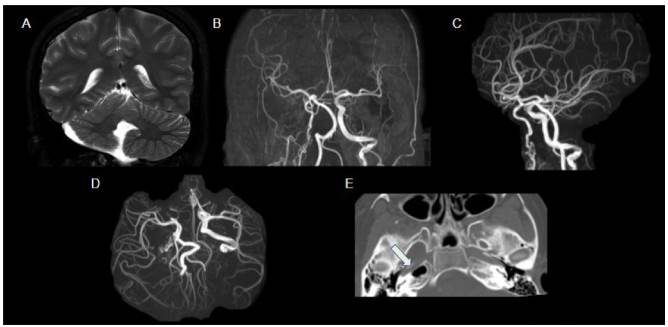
PHACE syndrome in 12-year-old female with a right-face hemangioma. Coronal T2WI (A) shows asymmetry of cerebellar hemispheres with volumetric reduction of the right hemisphere and hypoplastic vermis. MRA (B, C and D) shows thinning of the right internal carotid artery and multiple tortuous and irregular ipsilateral vessels (rete mirabile). CT of the skull base (E) depicts narrowing of the right internal carotid canal (arrow), thus signaling hypoplasia.
